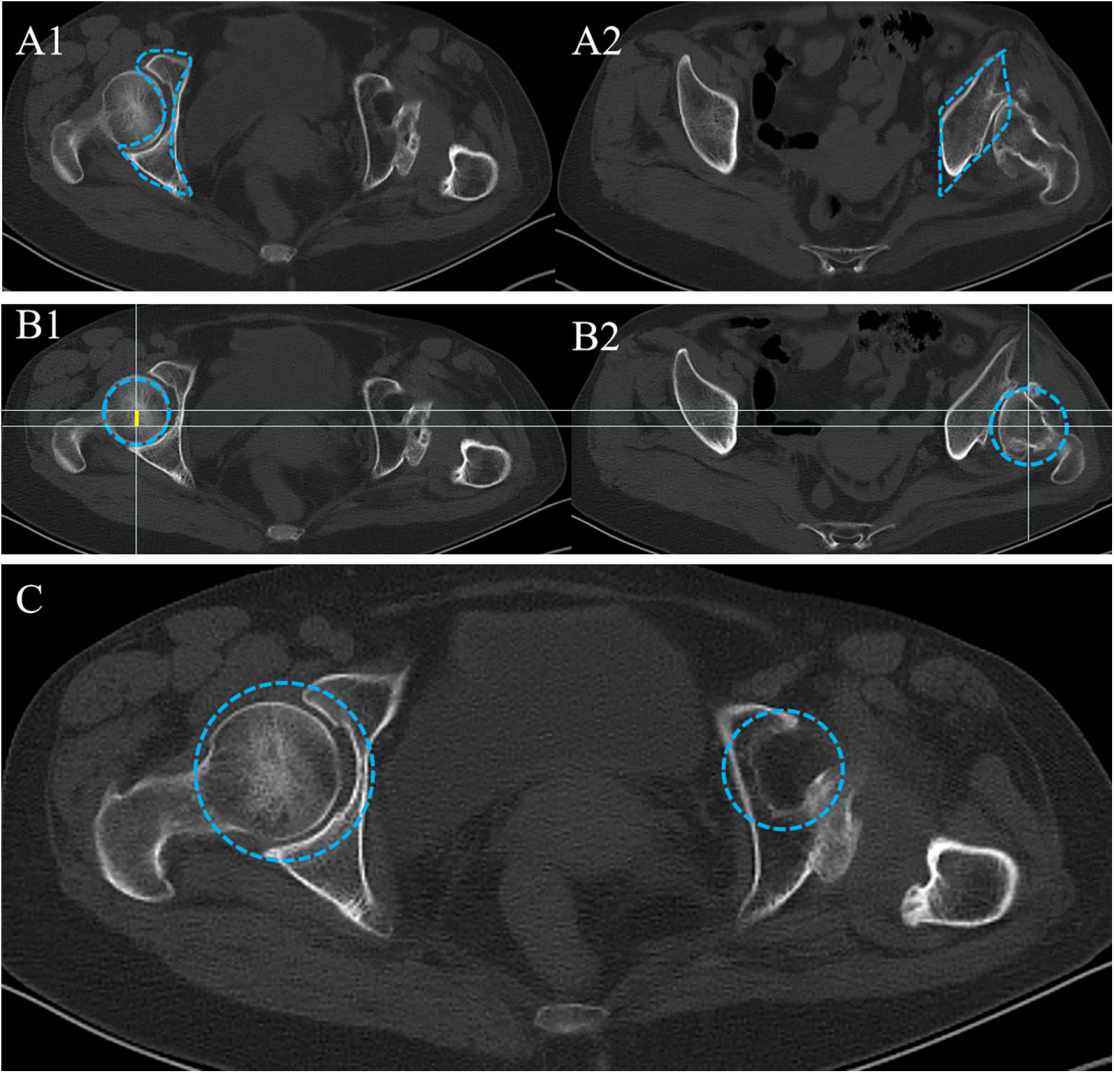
Axial CT radiological characteristics in patients with long-term unreduced hip joint dislocation. (A1) On the healthy side, the circumference of the femoral head on axial CT consists of the anterior, medial, and posterior columns of the acetabulum together into a region resembling a capital letter “I.” (A2) On the affected side, the area immediately adjacent to the femoral head is the ilium, which consists of the ilium, the false acetabulum, and the posterior wall of the original acetabulum around the posteriorly dislocated femoral head in an approximately rhomboid-like area called the rhomboid sign. (B) The patient's axial CT was placed in a standard grid line. The circular area enclosed by the blue dotted line was the affected side femoral head (B1) and the healthy side femoral head (B2). The yellow line indicates the distance of the rotation center of the affected side femoral head relative to the healthy side posteriorly. (C) The position of the ipsilateral original acetabulum allows a semicircular area, which is mirrored with the healthy acetabulum in a relatively small to healthy ratio, which is an image of an atrophic acetabulum on the affected side.
